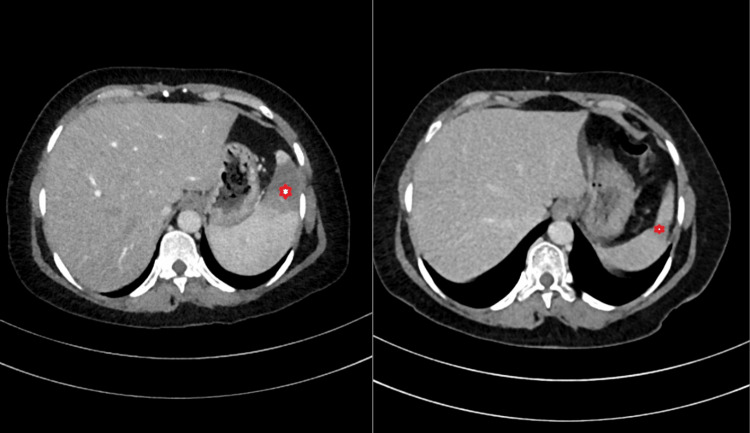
Contrast-enhanced CT of the abdomen. Left panel: Anterior splenic infarct pre-treatment. Right panel: Resolution of the splenic infarct after six weeks of appropriate antibiotic therapy.
